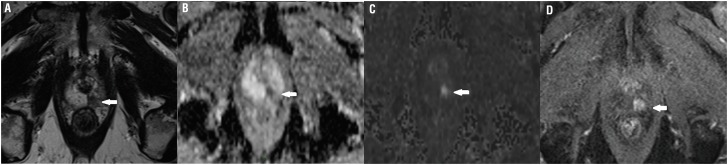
Seventy three years old man with PSA level of 3.4 ng/mL and normal DRE. MpMRI shows a 7 mm nodule in the left apical peripheral zone (T2-weighted imaging on A), with marked restricted diffusion seen on ADC map (B) and on b-value of 1500 (C). The lesion was categorized as very high suspicion for CS PCa (category 5) for both readers in both reading sessions despite the small size and DCE sequence. Early enhancement of the lesion is seen on DCE (D). Biopsy confirmed Gleason 4+4.
